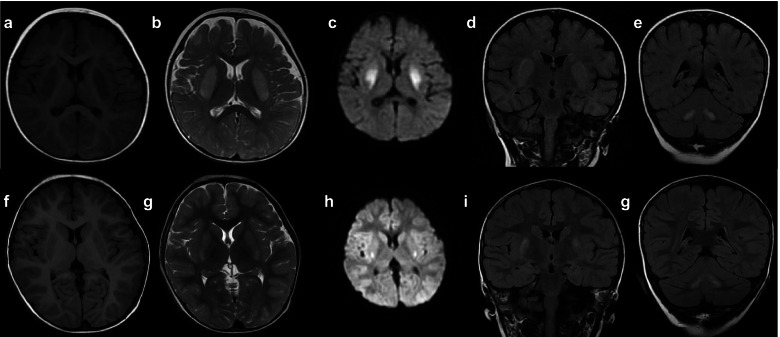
MRI of the brain during the first episode (a-e) and the second episode (f-g). af T1WI; bg T2WI; ch DWI; deig T2FLAIR. It showed the abnormal signal in bilateral caudate nucleus, lentiform nucleus, and dentate nucleus
